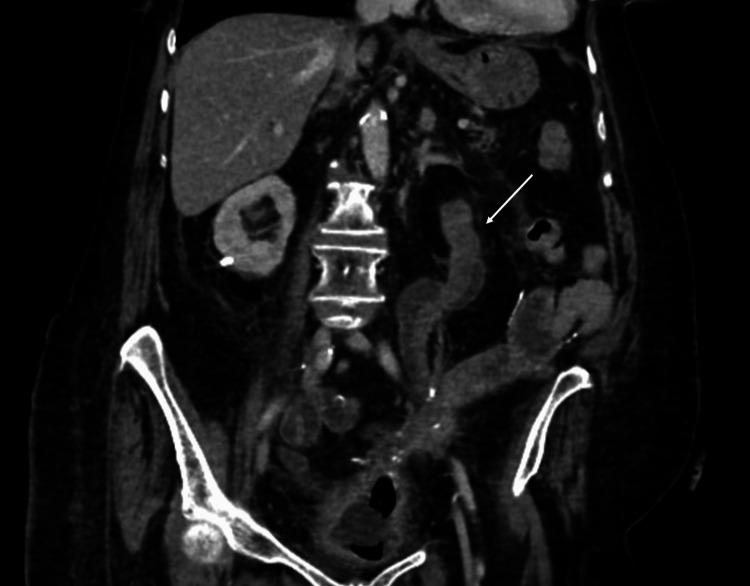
Coronal delayed-phase CT Coronal delayed-phase CT image of the abdomen demonstrating contrast extravasation into a dilated ureter (arrow), consistent with ureteroarterial fistula
